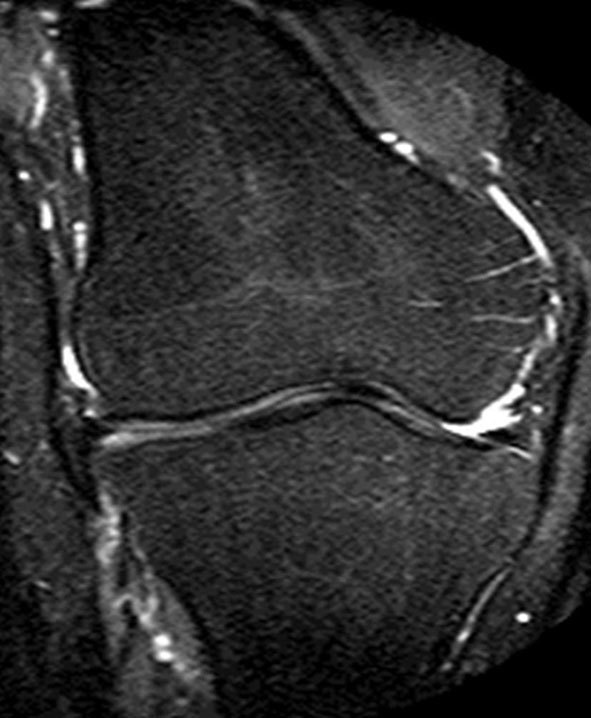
Coronal proton density fat-saturated MRI shows a hypoplastic tibial spine covered with cartilage.
